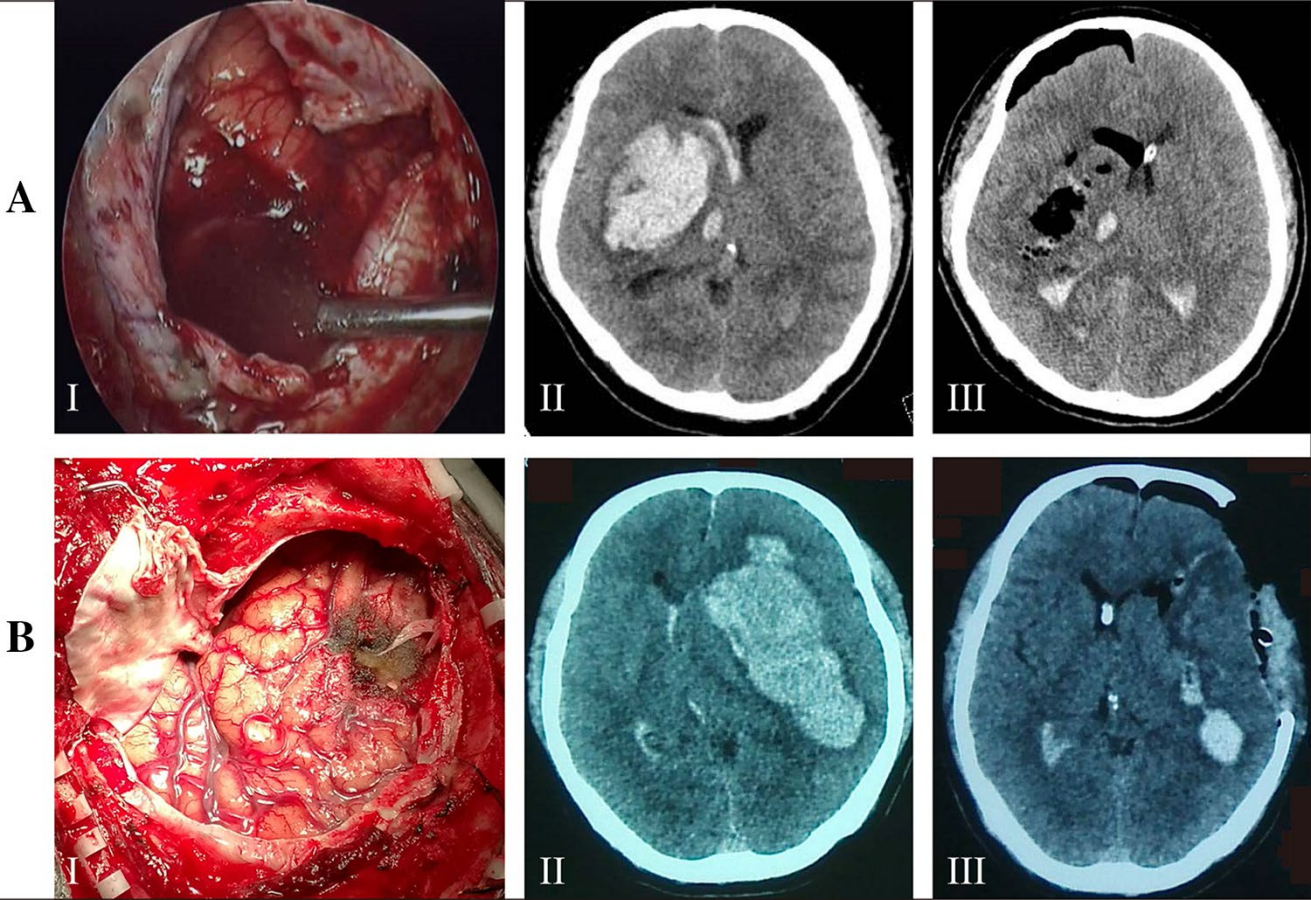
a Endoscopic surgery for large putaminal ICH without DC. The brain tissue collapsed immediately after endoscopic hematoma evacuation (I). Preoperative CT shows right hemispheric large ICH (II), and postoperative CT shows well-evacuated ICH with slight edema (III). b DC with hematoma evacuation for large putaminal ICH. A large frontoparietal craniectomy is performed, and hematoma is evacuated by endoscope (I). Preoperative CT shows left hemispheric large ICH (II), and postoperative CT shows the satisfactorily evacuated ICH, large left frontoparietal craniectomy, and a cannula in right ventricles (III)
